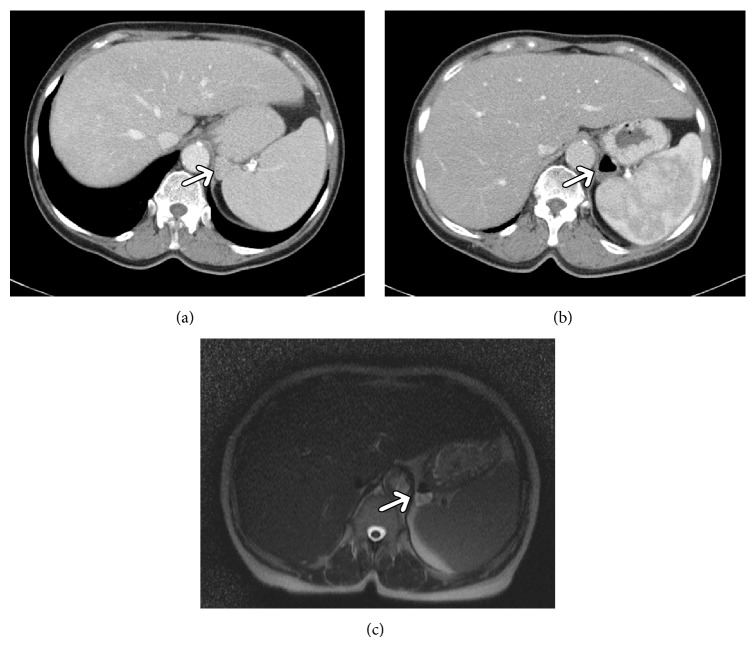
Imaging findings in a 70-year-old woman with known history of hepatocellular carcinoma. (a) Primary computer tomographic scan detecting a solid mass between the stomach aorta and spleen (arrow). The lesion was interpreted as an accessory spleen. (b) On computer tomographic scan 5 months after the primary investigation the lesion is cystic with air fluid level (arrow). (c) MRI image of the lesion (arrow).
